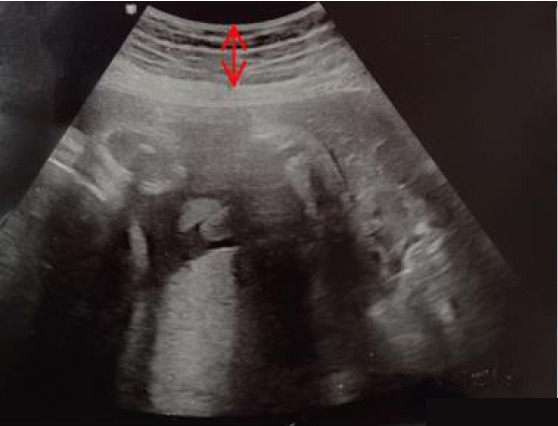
Axial ultrasound image. Arrow indicates skin-subcutaneous fat thickness.
